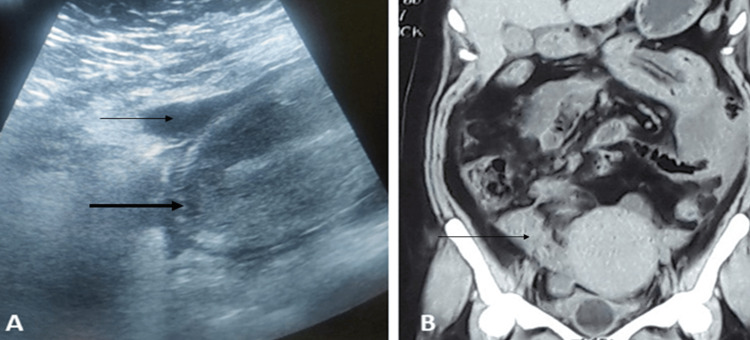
Preoperative imaging (A) Gray-scale ultrasound showing a mildly bulky uterus with an intact uterine contour (thick arrow) and the presence of ascites (thin arrow). (B) Contrast-enhanced CT of the abdomen showing multiple pockets of fluid collection (arrow) in the pelvis, suggestive of peritonitis.
